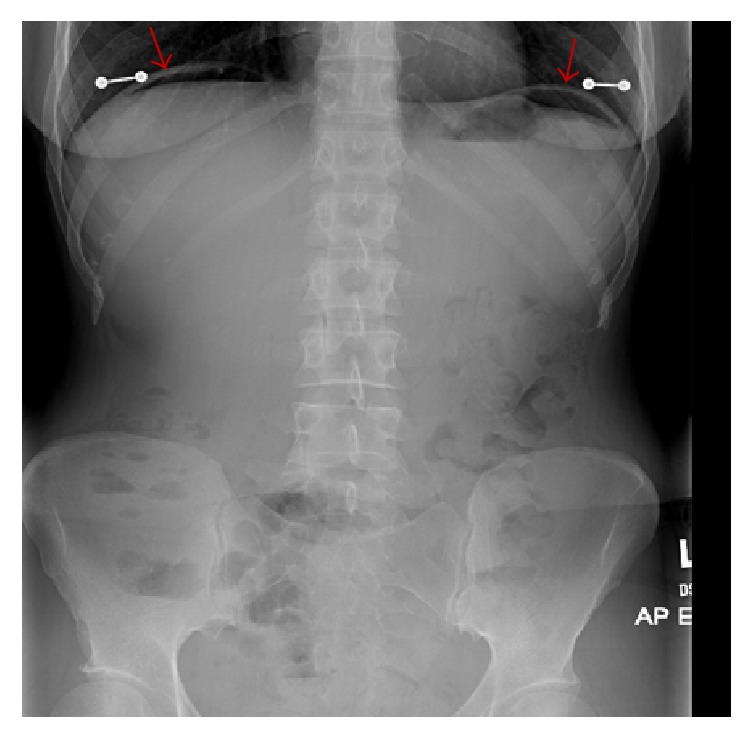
An anterior-posterior (AP) X-ray of the abdomen read as “free intraperitoneal air suggestive of a perforated viscus” (arrows).
